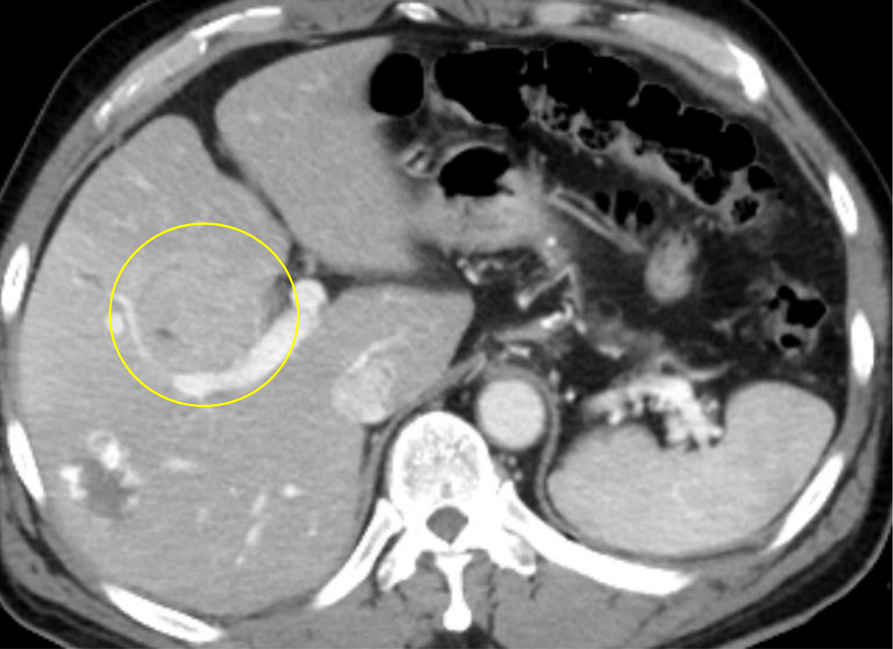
Preoperative abdominal computed tomography showed a 50 × 48 mm hepatic mass that pressed the anterior Glisson
